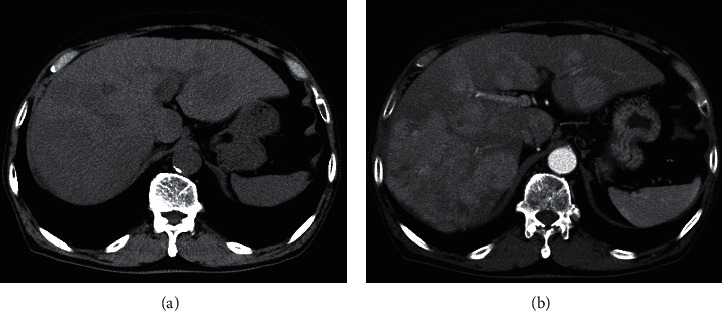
Nonenhanced computed tomography (a) and arterial phase of CT (b). Multiple liver tumors are depicted as low attenuation areas in nonenhanced CT and exhibited early enhancement in the arterial phase. These tumors were considered hepatocellular carcinomas.
